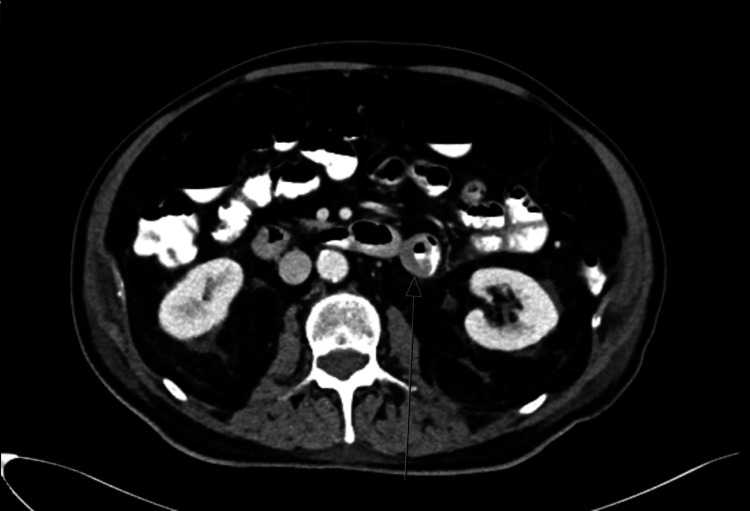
CT scan demonstrating a circular filling defect in the proximal jejunum, raising suspicion that this may be the source of the gastrointestinal bleeding.
